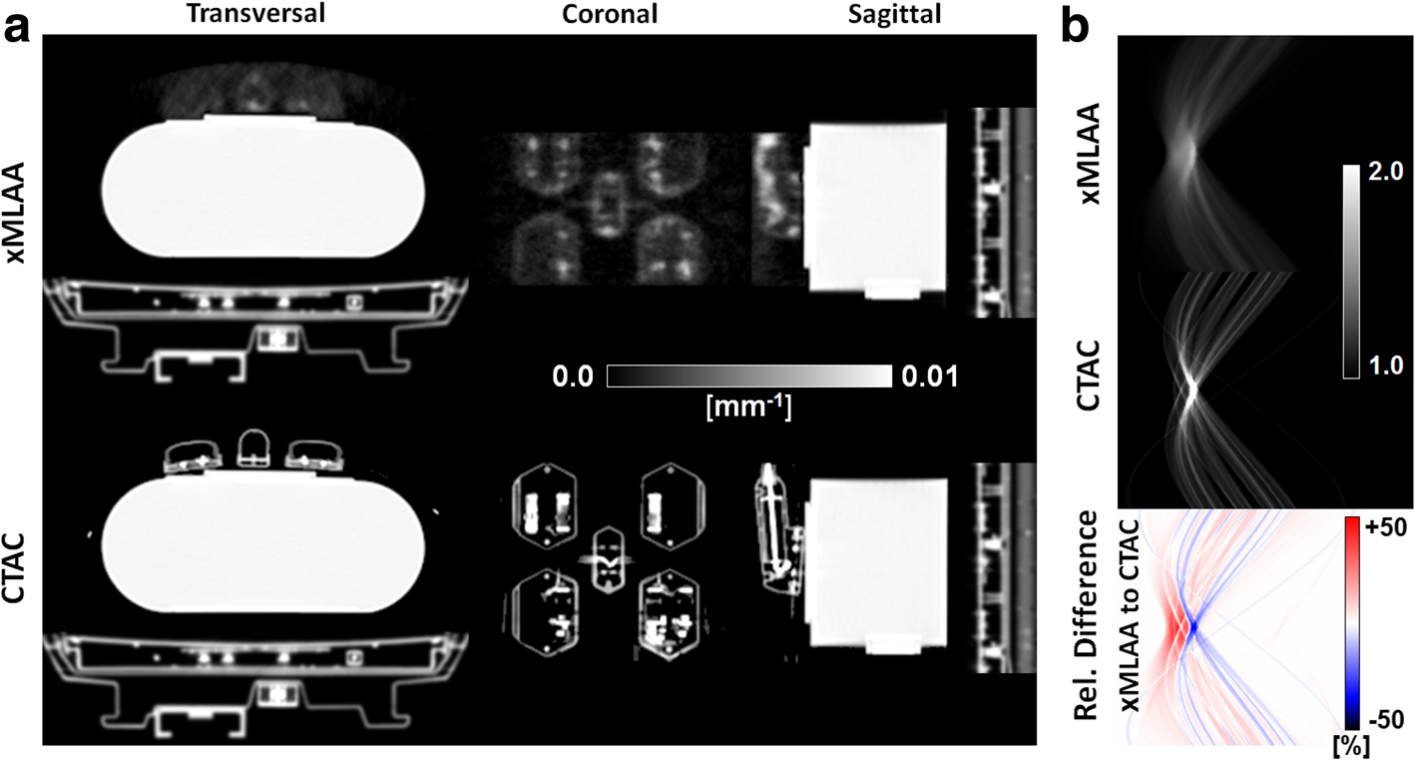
Results for the RF experiment: attenuation. a Transversal, coronal, and sagittal views of the xMLAA- and CT-based attenuation maps. b Attenuation correction factors (ACFs) of the RF coil only. The sinograms correspond to the same transversal plane as shown in (a)
